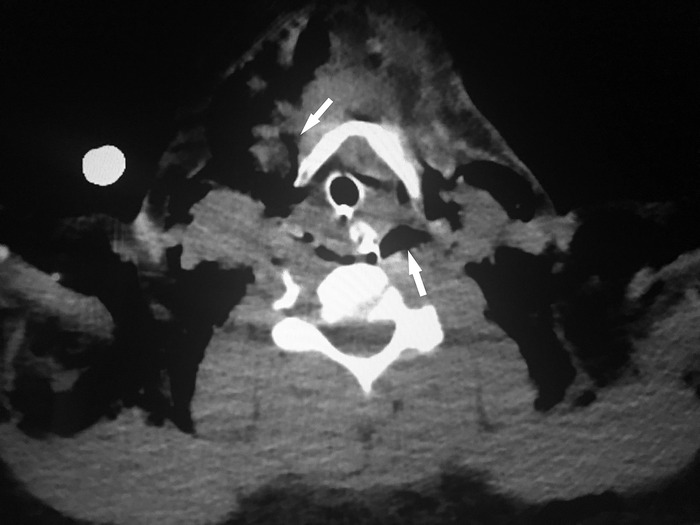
Computed tomography of the neck shows subcutaneous air in the anterior and posterior compartments of the neck (white arrows).
